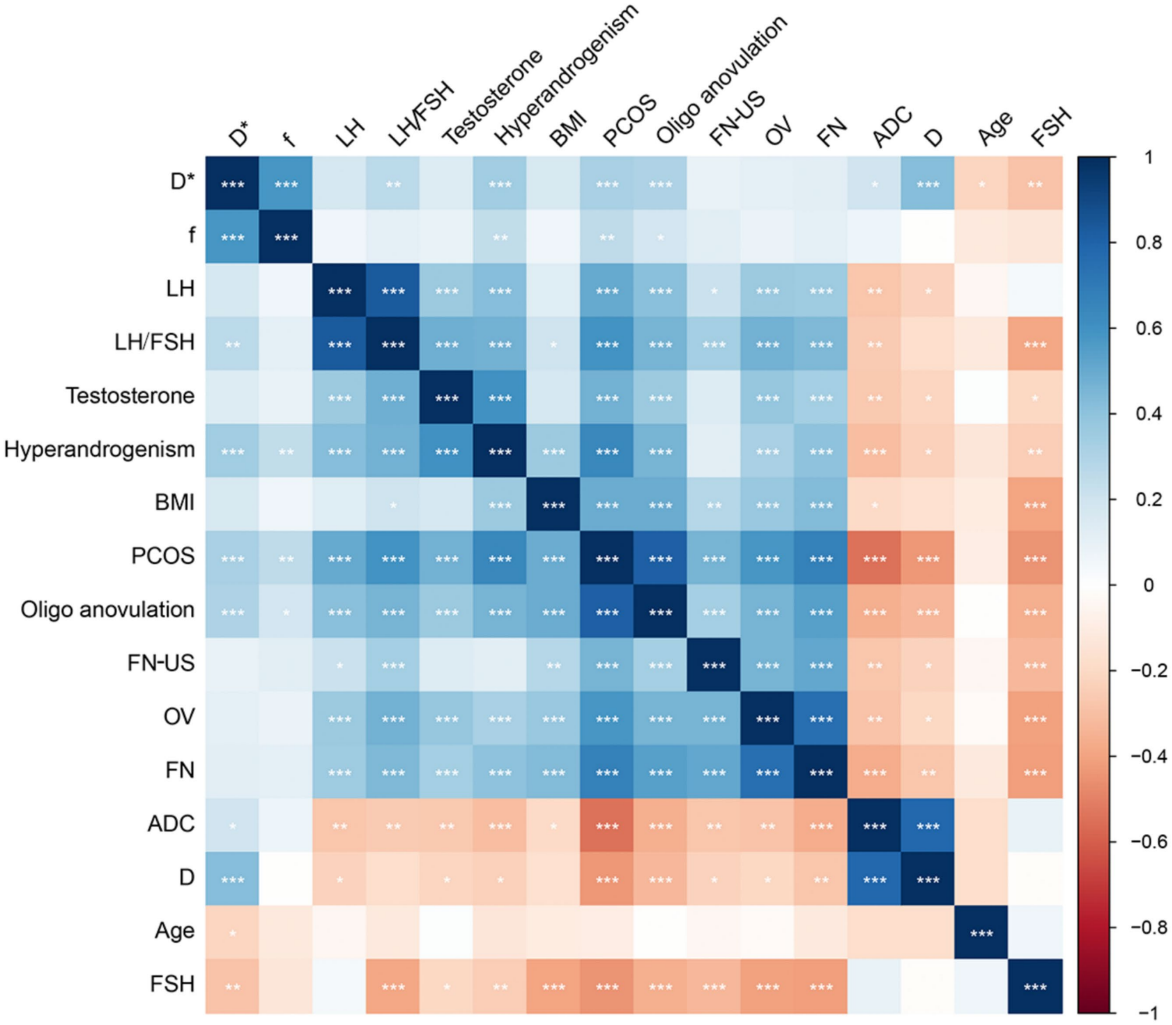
Correlation heatmap between morphological parameters and functional parameters. The color intensity in the heatmap indicates the strength and direction of the correlation between the parameters. Darker colors typically represent stronger correlations, whether positive (blue) or negative (red). Asterisks on the heatmap cells denote the significance of the correlation. ^*^*p* < 0.05; ^^*p* < 0.01; ^*^*p* < 0.001. BMI, body mass index; FN, follicle counted on MRI; OV, ovarian volume calculated on MRI; FN-US: follicle count by ultrasound; ADC: apparent diffusion coefficient; D, true diffusion coefficient; D*, pseudo-diffusion coefficient; f: perfusion fraction; LH, luteinizing hormone; FSH, follicle stimulating hormone; IVIM, intravoxel incoherent motion; PCOS, polycystic ovary syndrome.
